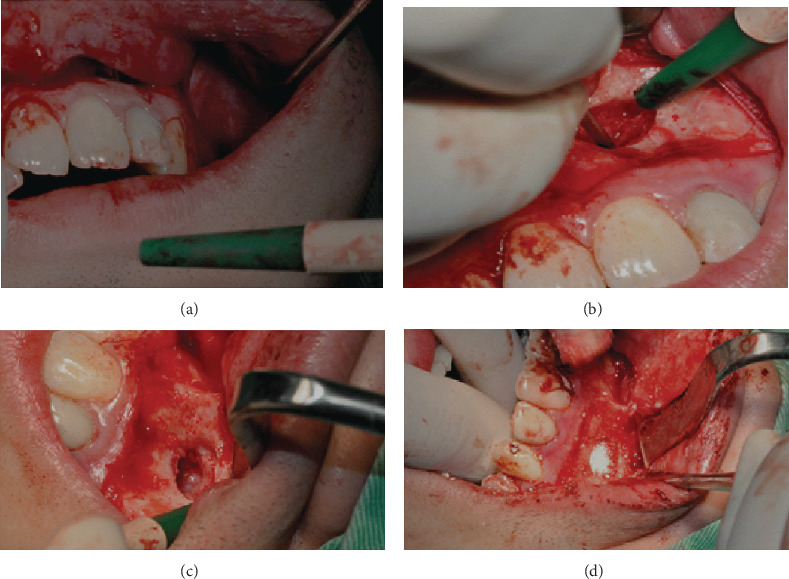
Periapical surgery procedure of tooth 22: (a) flap preparation, (b) enucleation of lesion, (c) bone preparation for graft, and (d) bone graft placement.
